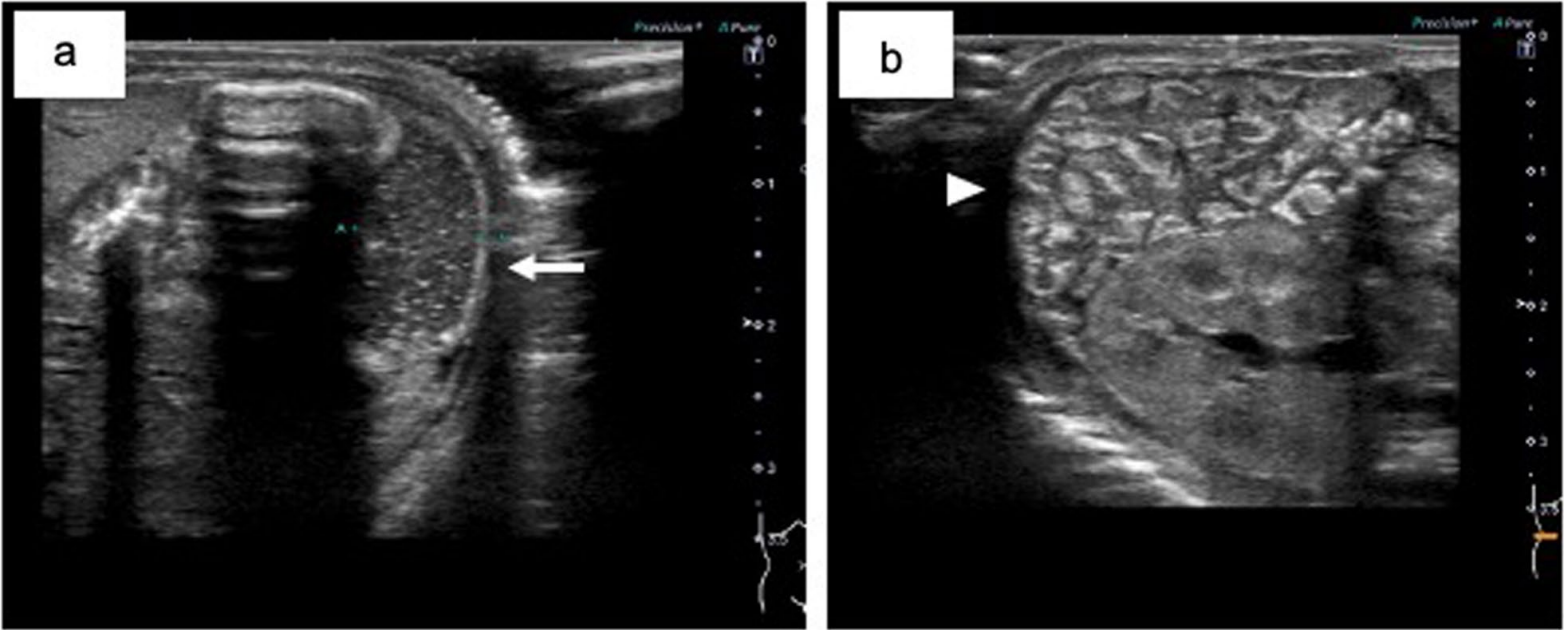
Abdominal ultrasonography findings. A dilated jejunum (arrow) (a) and collapsed small intestine (arrowhead) (b) are seen
